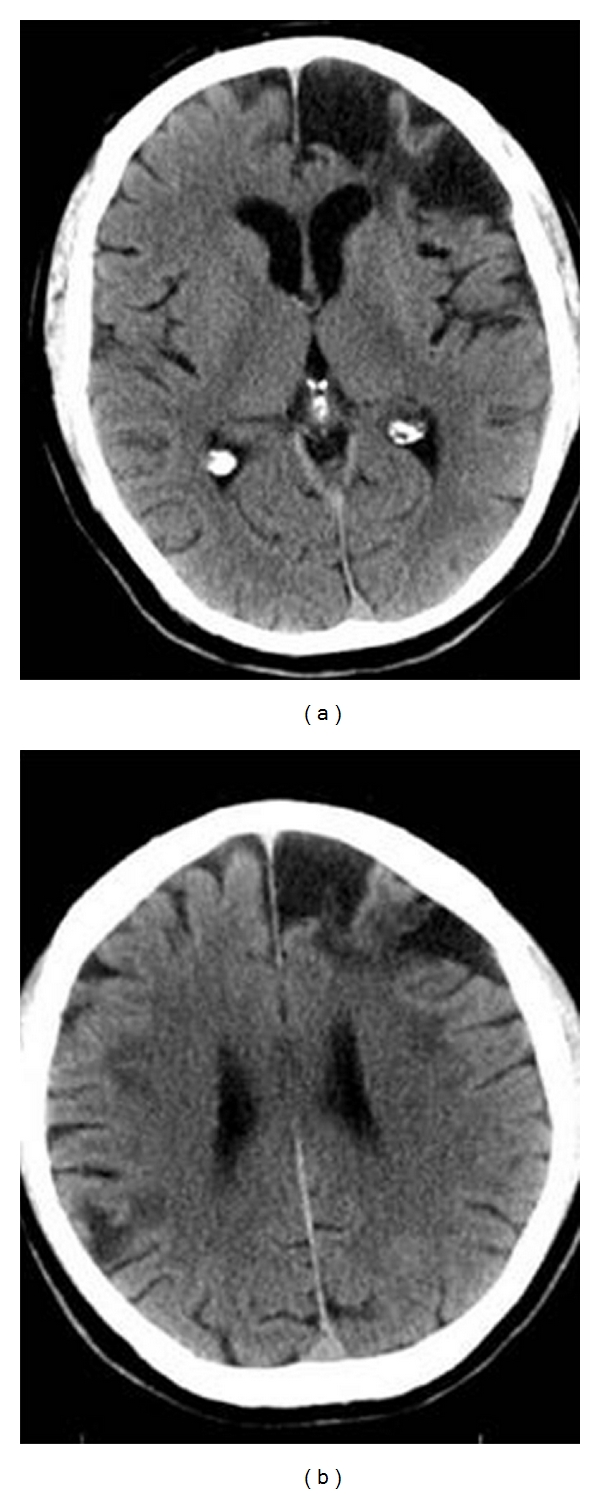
Participant no. 2 with a left frontal lobe lesion. The CT image in (a) depicts the extensive left inferior frontal lobe lesion occurring as a result of an anterior communicating artery aneurysm. The CT image in (b) depicts the same lesion extending contiguously to include the infarcted tissue in the left anterior superior frontal lobe.
